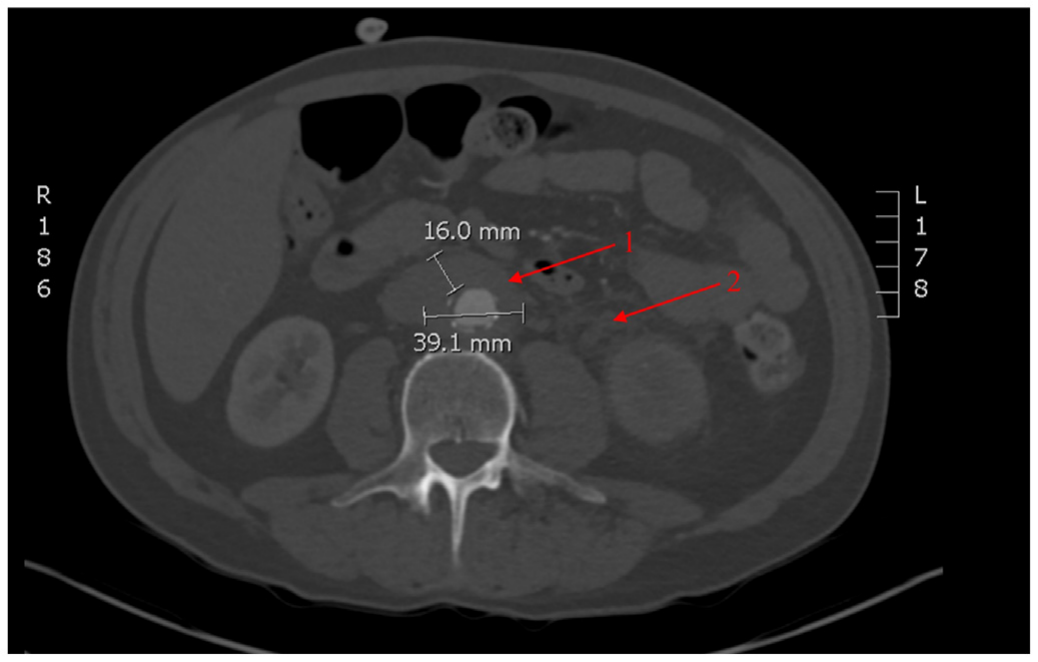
Computed tomography (CT) angiography of the abdomen from April 2022. One month after the discontinuation of nivolumab. Infrarenal aortitis (1) with maximal aortic wall thickness measured at 16 mm and axial diameter 39.1 mm. New finding of retroperitoneal fibrosis (2).
